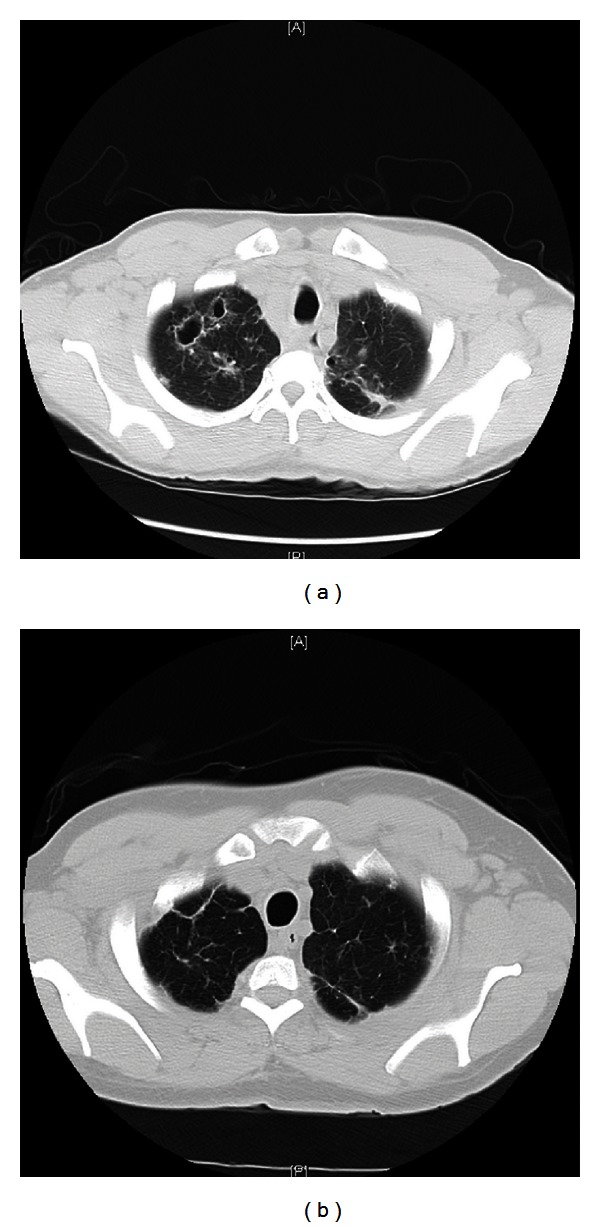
(a) CT scan of chest revealing cavitary lesions. (b) CT scan of chest shows resolution of cavitary lesions.
